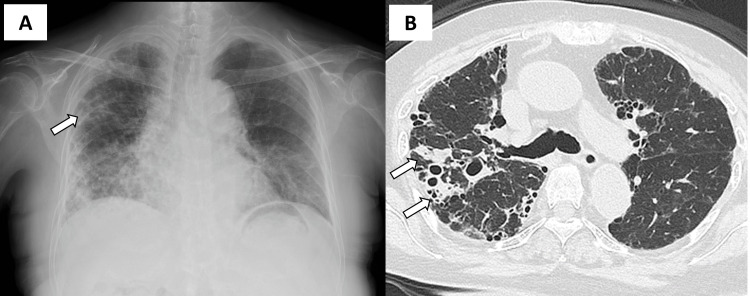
Chest X-ray and computed tomography images at the time of hospitalization The chest X-ray revealed newly developed consolidation in the right upper lung field (arrow) accompanied by pre-existing reticular shadows in the lower bilateral lung fields (A). Chest computed tomography (CT) demonstrated consolidation with bronchial wall thickening in the right upper lobe (arrow) (B).
